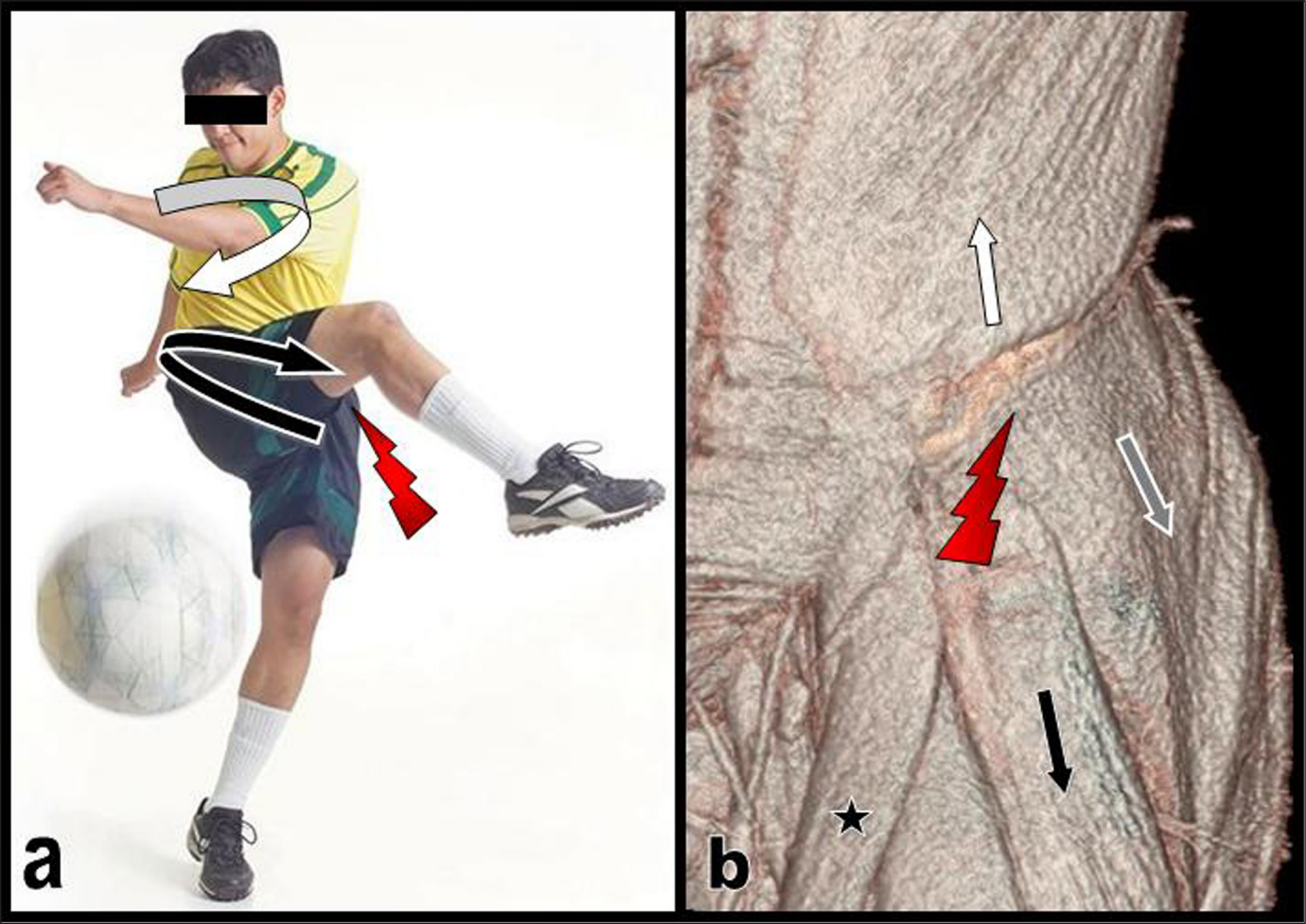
Typical posture of a right-handed soccer during fast kicking (a) volume rendering CT view of the muscles inserting on the iliac crest of our patient (b). Kicking is initiated by rotating the pelvis far to the left (curved black arrow on a) around the supporting left leg and by bringing forwards the thigh of the right kicking leg while maintaining the upper trunk to the right (curved white arrow on a). The sudden traction and contraction of the left external oblique (white arrow) probably pulls the apophysis off the left iliac crest. The action of the external oblique muscle is abruptly thwarted (red flash) by the antagonist traction of the gluteus medius (grey arrow) but merely of the tensor fascia lata (black arrow) that displaces the avulsed apophysis outwardly and downwardly. Black star = Sartorius.
